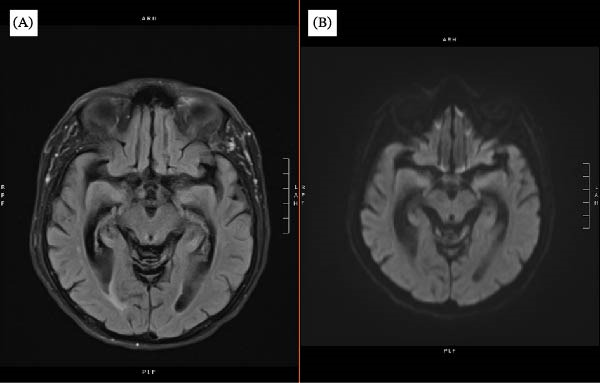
Admission brain MRI without definitive HSV‐1 encephalitis findings. (A) Axial T2‐FLAIR image and (B) axial diffusion‐weighted image (DWI) at the temporal lobe level demonstrate ventriculomegaly without clear mesial temporal, insular, or frontal lobe signal abnormality suggestive of HSV‐1 encephalitis.
